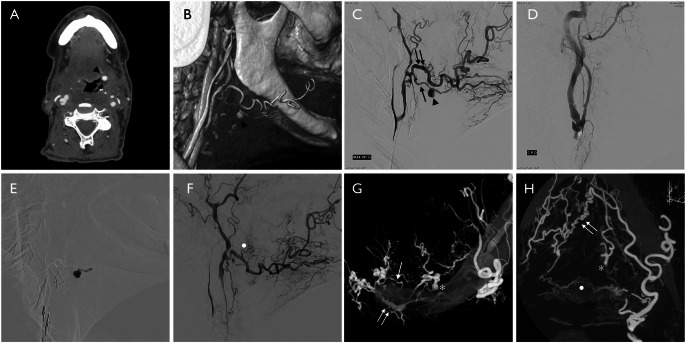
(A) Axial CTA, showing the left PLA close to the ipsilateral vallecula (*arrowhead*); (B) CTA 3D reconstruction showing the left PLA (*arrowhead*) in the medial portion of the suprahyoid region; (C) DSA, lateral view with injection of the left ECA showing the pseudoaneurysm (*arrowhead*) of the main trunk of the lingual artery (*arrow*) and the ipsilateral facial artery (*double arrow*); (D) DSA, lateral view with injection of the right ECA showing the absence of the ipsilateral facio-lingual trunk; (E) superselective injection of diluited Glue within the pseudosac and the distal portion of the parent artery; (F) DSA, lateral view with injection of the left ECA after embolization showing the complete occlusion of the PLA and the proximal part of the lingual artery; dorsal tongue arcade (*white dot*); G-H) XperCT frontal Gradient-shaded view (G) and axial MIP-reconstructions (H) showing the three arterial anastomotic arcades: (i) the submental arcade (*white double arrow*) between the two submental branches of the facial arteries, supplying the contralateral facial and lingual arteries; (ii) the sublingual arcade (*white arrow*) between the two sublingual branches of the lingual arteries supplying the left lingual artery and (*iii*) the dorsal tongue arcade (*white dot*) between the two dorsal branches of the lingual arteries. The asterisk points the distal segment of the left lingual artery
